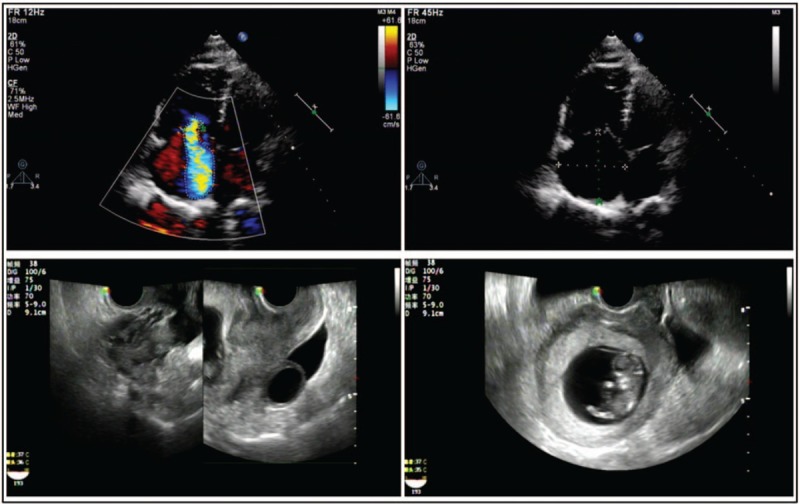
Ultrasonography on admission. Moderate-to-severe tricuspid regurgitation, and a distended right ventricle and atrium were revealed by the transthoracic echocardiography before thrombolysis. The fetal Doppler instrument revealed fetal heart 2 h after thrombolysis.
